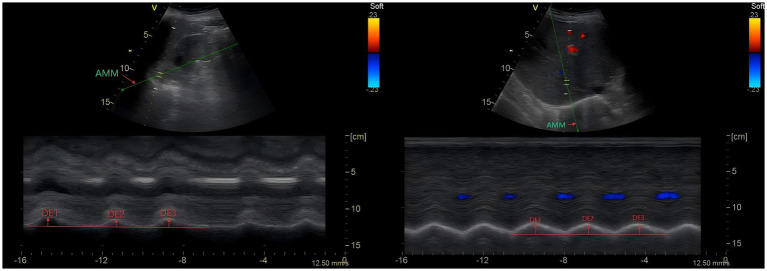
Diaphragm ultrasonography1: DE of left and right hemidiaphragm.
